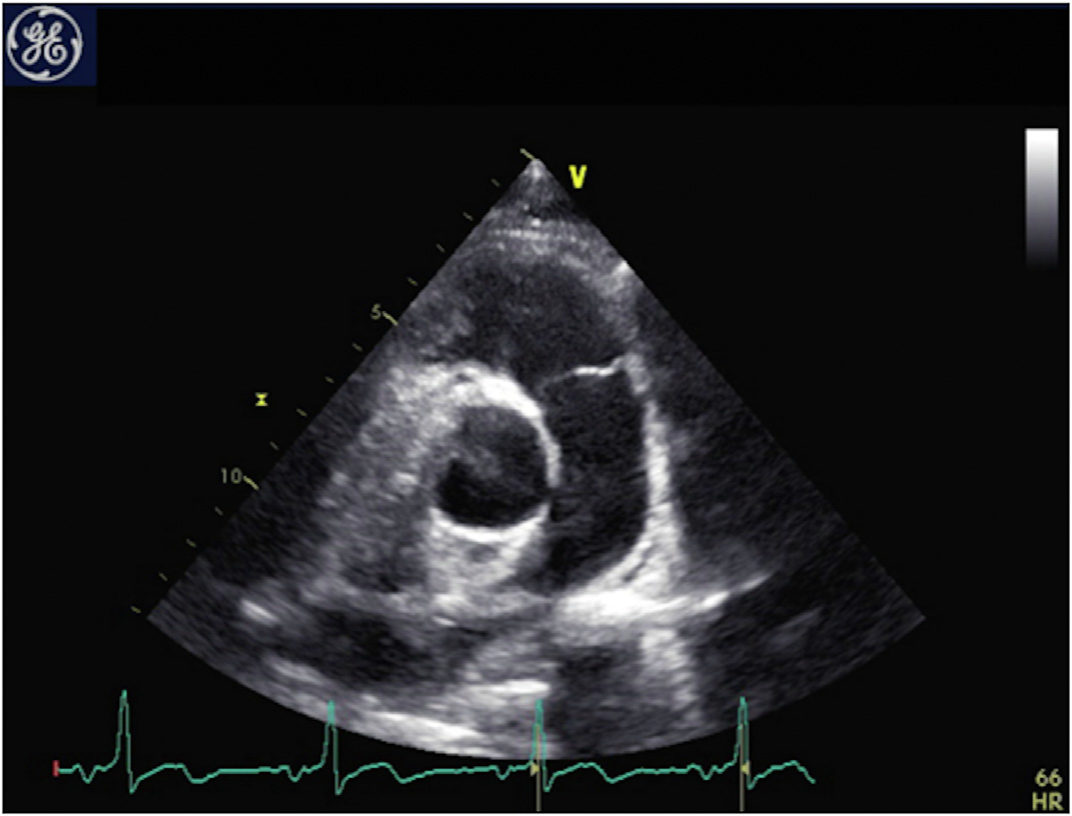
Echocardiography Parasternal short-axis view showing the absence of the left pulmonary artery.
